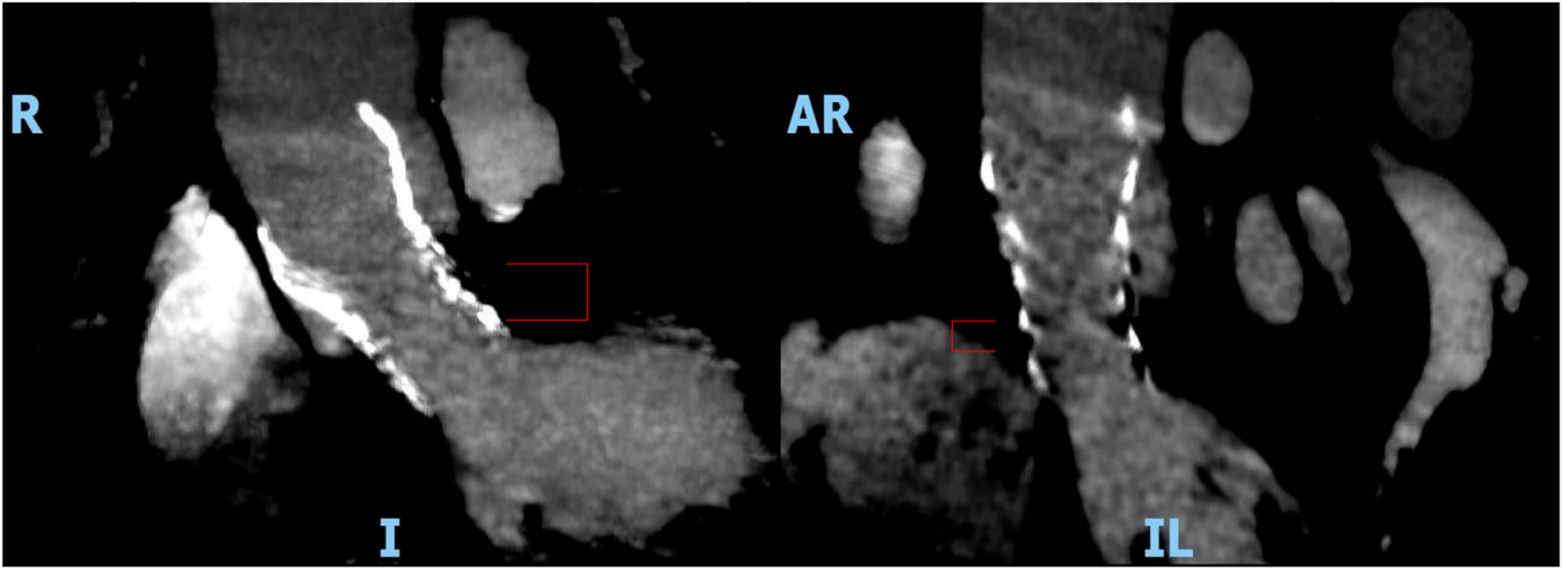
Post‐procedure CT image. Subannular positioning of the TAVI valve, anastomosis between autograft and native LVOT is the landing zone (annotated by the red third bracket). [Color figure can be viewed at wileyonlinelibrary.com]
